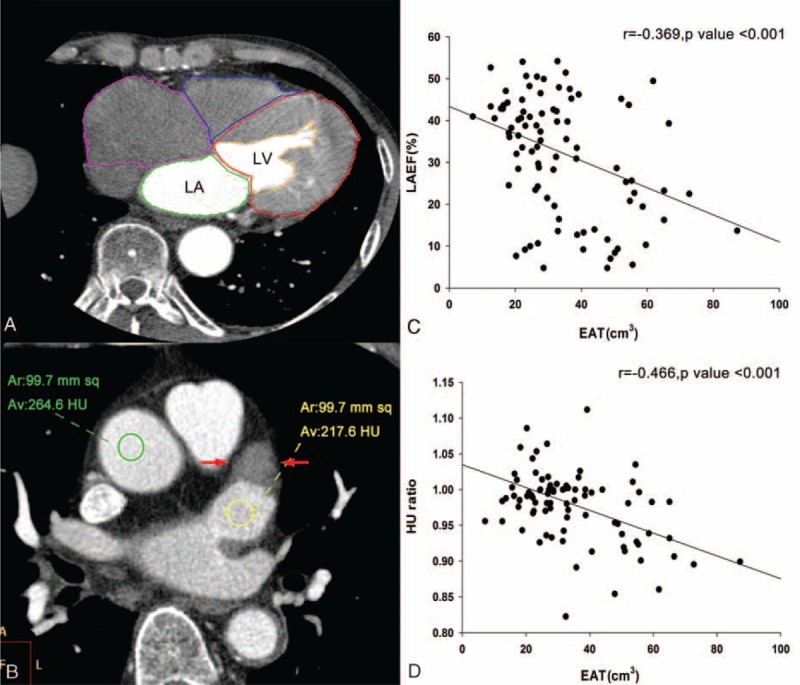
(A) The method used to evaluate left atrium (LA) and left ventricle function by tracing the endocardial border using multidetector computed tomographic images. (B) The method used to acquire the HU ratio of the LA appendage (LAA) and ascending aorta (AA). A 1-cm^2^ region of interest was sampled within the LAA to select the 1-cm^2^ region showing the lowest HU value. However, sampling within the filling defect of the LAA (between arrows) was avoided. At the same level, another 1-cm^2^ region was selected in the contrast-enhanced lumen of the AA. (C) LA emptying fraction (EF) was negatively correlated with the abundance of epicardial adipose tissue (EAT). (D) LAA/AA HU ratio was negatively correlated with the abundance of EAT.
